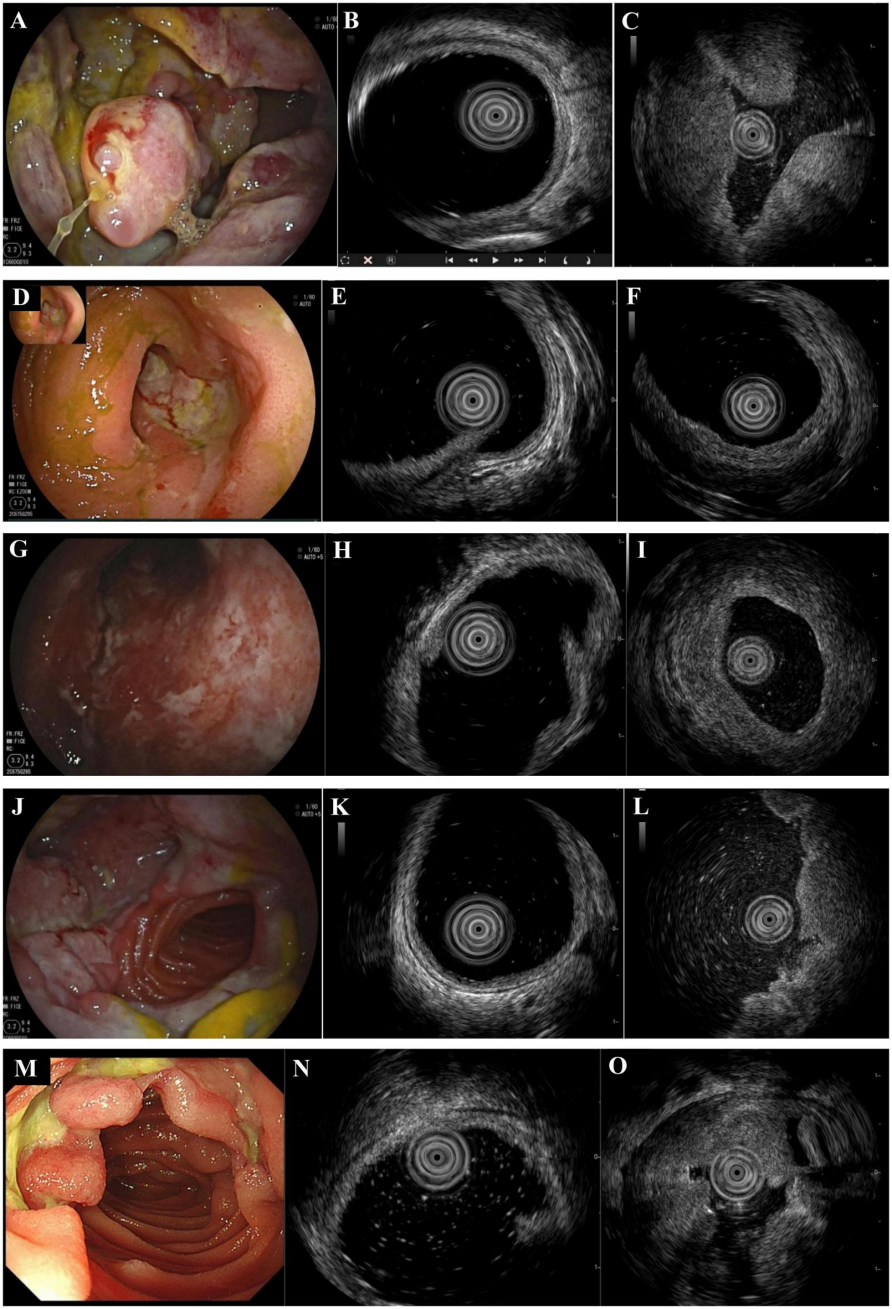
Clinical data of five cases included in this study. (A) DBE shows a bulging, irregular mass ∼10 cm long in the distal jejunum; the surface of the mass is covered with white mucus (Case 1). (B) Normal small intestinal wall on EUS shows no thickening of the intestinal wall (Case 1). (C) EUS shows thickening of the entire bowel wall, fusion and loss of demarcation of bowel wall layers, hypoechoic changes in some areas, and even lower echogenicity inside the hypoechoic areas (Case 1). (D) DBE shows a longitudinal lamellar ulcer with a thick white coating and congestion of the mucosa surrounding the ulcer in the proximal ileum (Case 2). (E) Normal small intestinal wall on EUS shows no thickening of the intestinal wall (Case 2). (F) EUS shows thickening of the entire intestinal wall, indistinct demarcation between the mucosal and submucosal layers in some areas, and localized hypoechoic or hyperechoic changes in the submucosa and muscularis propria (Case 2). (G) DBE showed a bulging, circumferential mass ∼15 cm long with a white coating and congestion in the proximal ileum (Case 3). (H) Normal small intestinal wall on EUS shows no thickening of the intestinal wall (Case 3). (I) EUS shows thickening of the entire bowel wall, fusion of bowel wall layers, indistinct demarcation between the submucosa and muscularis propria, hypoechoic changes in some areas of the submucosa, and even lower echogenicity inside the hypoechoic areas within muscularis propria (Case 3). (J) DBE shows an irregular, elevated, circumferential mass ∼10 cm long with a yellowish-white coating (Case 4). (K) Normal small intestinal wall on EUS shows no thickening of the intestinal wall (Case 4). (L) EUS shows that the entire bowel wall is thickened, and there is fusion and loss of demarcation of bowel wall layers (Case 4). (M) DBE shows multiple irregular, depressed, debris-covered ulcers starting from the distal duodenum to the proximal jejunum (Case 5). (N) Normal small intestinal wall on EUS shows no thickening of the intestinal wall (Case 5). (O) EUS shows that the entire bowel wall is thickened, predominantly in the muscularis propria (Case 5). DBE = double balloon enteroscopy, EUS = endoscopic ultrasonography.
